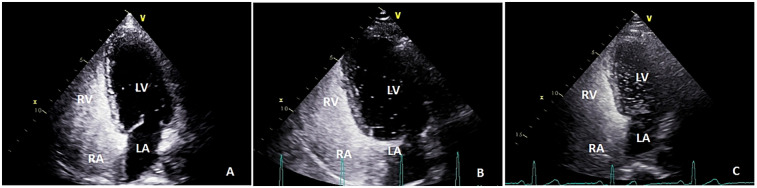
The number of microbubbles in left atrium (right-to-left shunt) during the five cardiac cycles after right atrial echocardiography was observed by transthoracic apical four-chamber view: (A) small (<10 microbubbles); (B) medium (10–30 microbubbles); and (C), large (>30 microbubbles); LA, left atrium; RA, right atrium; LV, left ventricle; RV, right ventricle.
